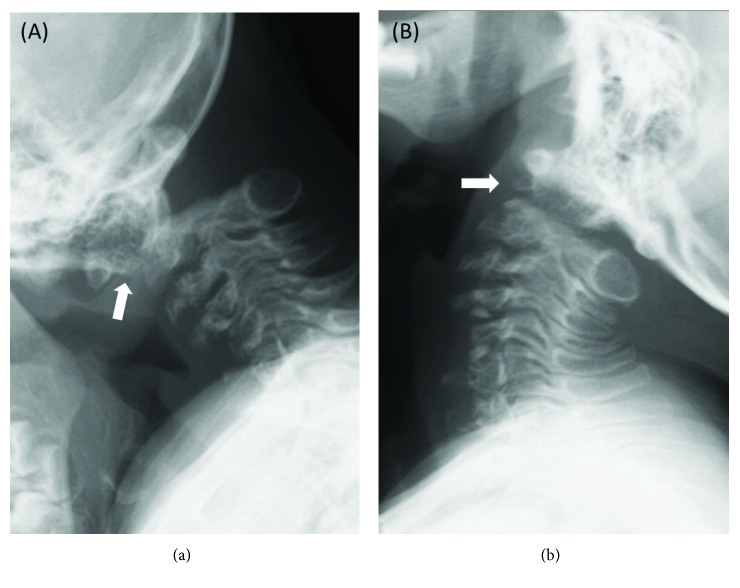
(a, b) Lateral plain radiographs of the cervical spine in flexion and extension at the age of 5 years. Multiple dysplastic vertebral bodies and atlantoaxial instability with os odontoideum are evident (*white arrows*).
